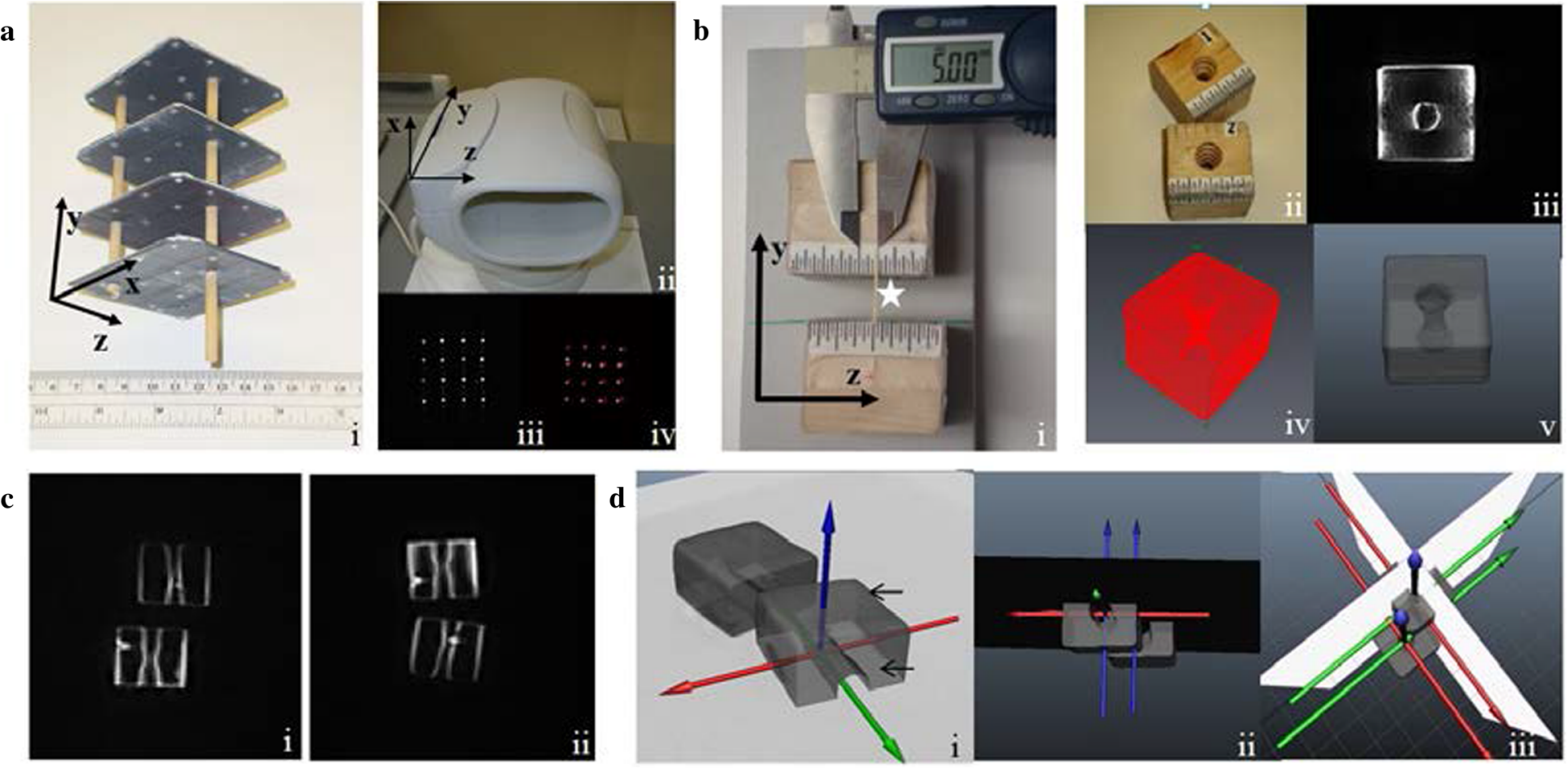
Overview of the animation processes leading to the quantification of a single-plane and biplanar displacements. a Imaging volume calibration: (i) the calibration grid with orientation of the plates in space, (ii) MRI coil with orientation of the imaging volume, (iii) & (iv) pre- and post-digitized bead images from the grid. b (i) Shows positioning of a translation trial. The solid-body models are spaced ~10 mm apart flat on a foam platform. The lower cube has been translated by 0.5 mm to the right relative to the upper cube, indicated by a wooden pointer (*asterisk*) and measured by the caliper. The orientation of the displacement has been shown by the coordinate axis. (ii) View of the wooden cubes. (iii) High-resolution axial T_1_ image slice through a cube. (iv) Representative 3-D model of a segmented cube. (v) Model as viewed after being imported into the animation environment. c (i) Representative image from a single-plane translation trial with the T_1_ sequence. (ii) Representative image from a single-plane rotation trial with the contrast-enhanced sequence. d (i) Representative single-plane rotoscoping “scene.” The image slice (off-white background) lies obliquely across the figure. The solid-body shadow is visible with its outline in the image slice (*lower arrow*). Upper half of the superimposed cube model is visible (*upper arrow*) with the anatomical axis. (ii) Image frame from a translation trial viewed from the top of the animation scene. The two cube models are cut through by the image slice (dark horizontal plane) across the hourglass holes within the models. (iii) Orthogonal image slices with registered 3D models
